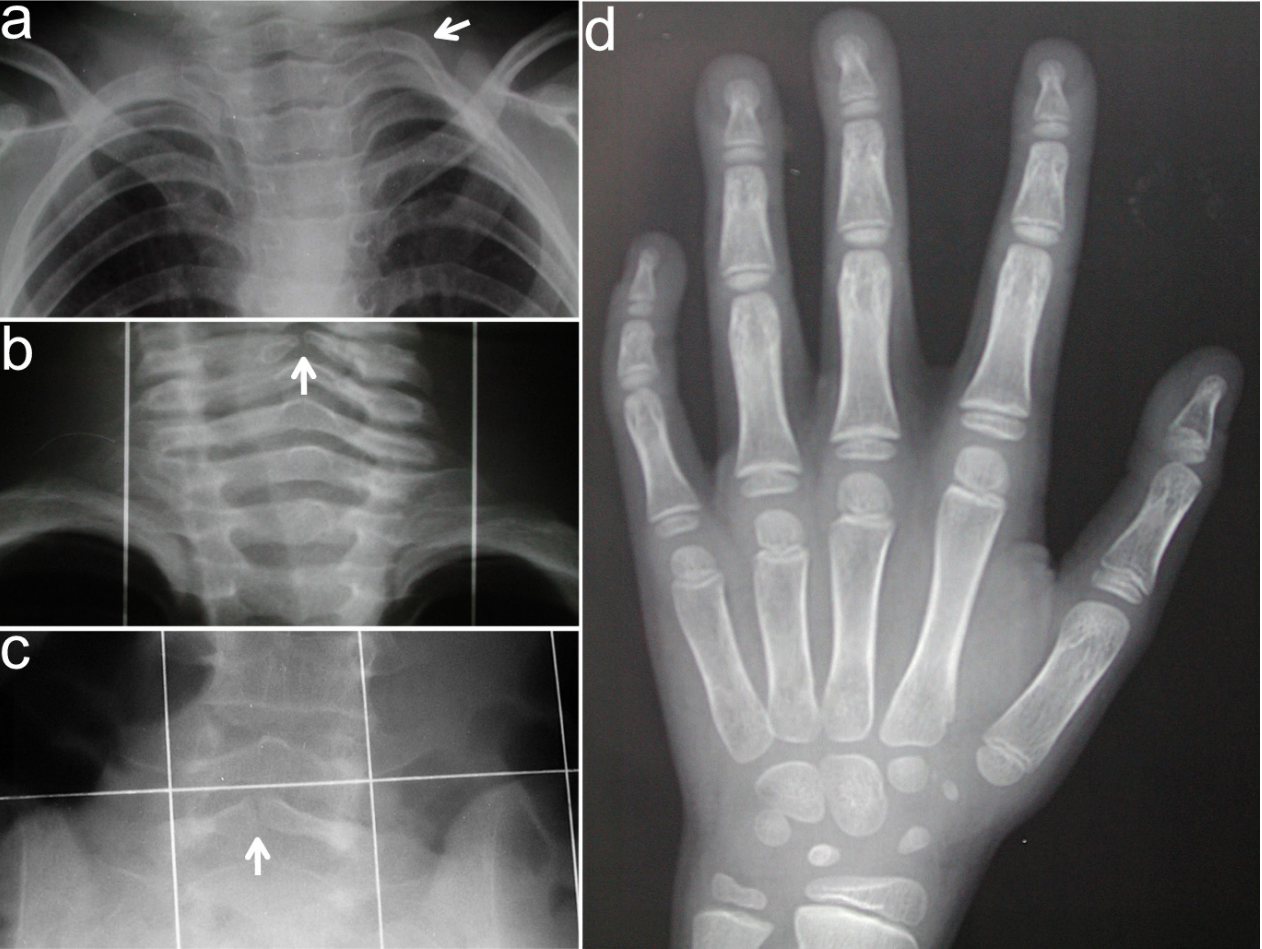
Common skeletal defects observed in KBG syndrome include supernumerary cervical rib (a, arrow), schisis of the posterior arch of cervical (b, arrow) and/or sacral (c, arrow) vertebrae. Left hand X-ray of a 10-year-old male KBG patient (d) showing shortened tubular bones especially of the III-IV-V metacarpus, the I distal and the V middle phalanges with clinodactylous V finger. Bone age is delayed in particular with respect to carpal bones.
